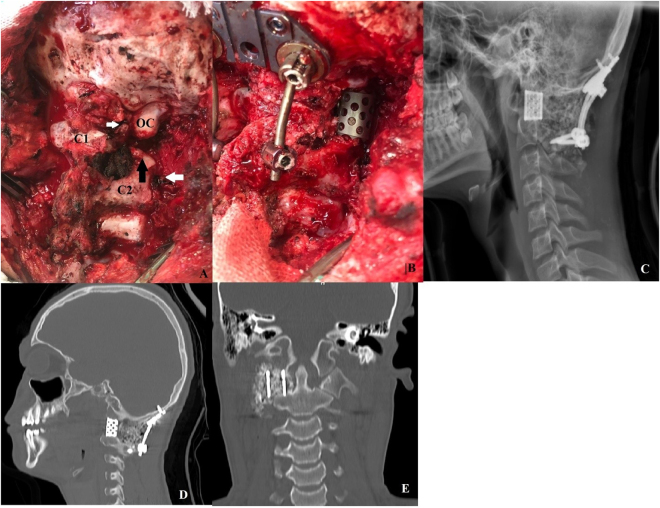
A: the intraoperative view shows a cavity due to total resection of the tumor (OC: occipital condyle, C1 the remaining of the atlas posterior arc after tumor resection, C2, and black arrow: the superior articular surface of the axis, white arrows: proximal and distal part of the VA that was ligated). B: after drilling and occipitocervial fixation, an appropriately sized mesh cage was fixed between the axis articular surface and the occipital condyle. C: postoperative lateral plain X-ray showing evidence of reconstruction, D and E: sagittal, and coronal view on CT scan demonstrating the mesh cage between the axis articular surface and the occipital condyle.
